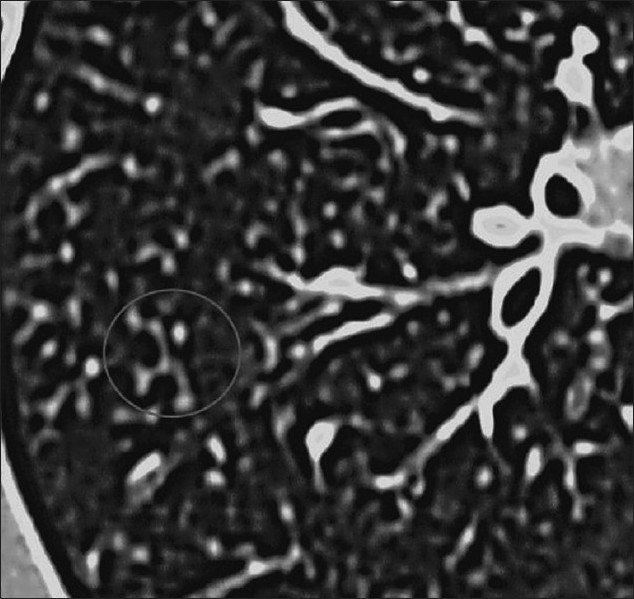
Mycobacterium tuberculosis*. High-resolution computed tomography shows a tree-in-bud appearance
